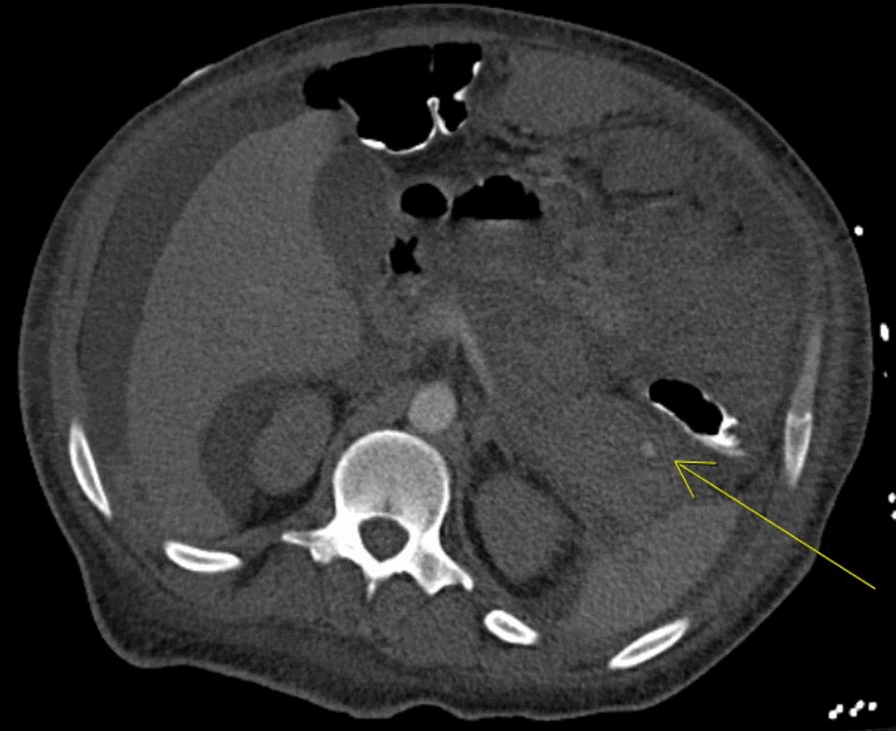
CT angiography of the Abdomen. A 7 mm arterially enhancing focus in the tail of the pancreas that follows the blood flow (Arrow), consistent with a pseudoaneurysm, with an associated large left upper quadrant hematoma and small amount of hemorrhage in the right paracolic gutter
